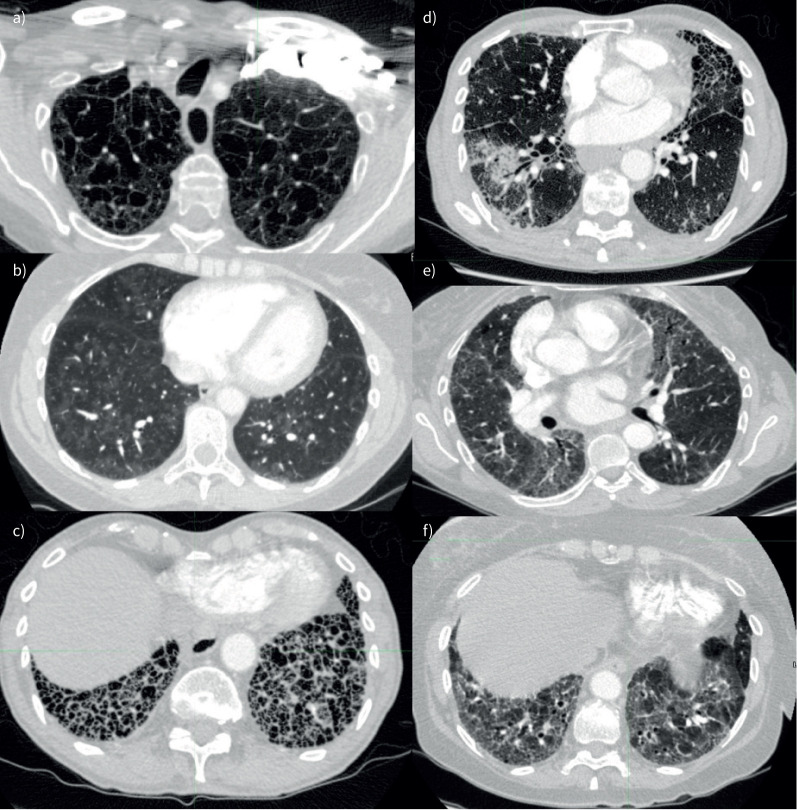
Computed tomography lung parenchymal patterns assessed. a) Emphysema, b) centrilobular ground glass change (windowed to emphasise subtle pattern), c) honeycombing, d) consolidation (with surrounding ground glass change), e) ground glass change and f) fibrosis.
